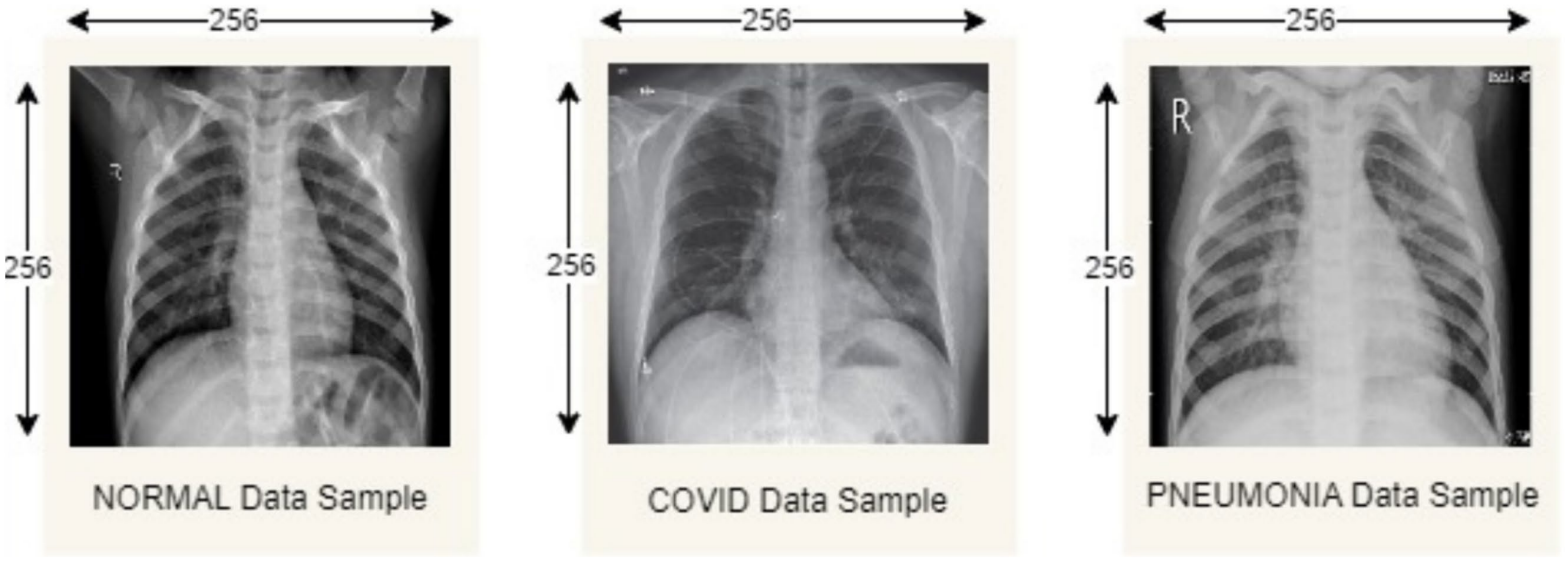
Normal, COVID19, pneumonia chest X-ray image samples.
